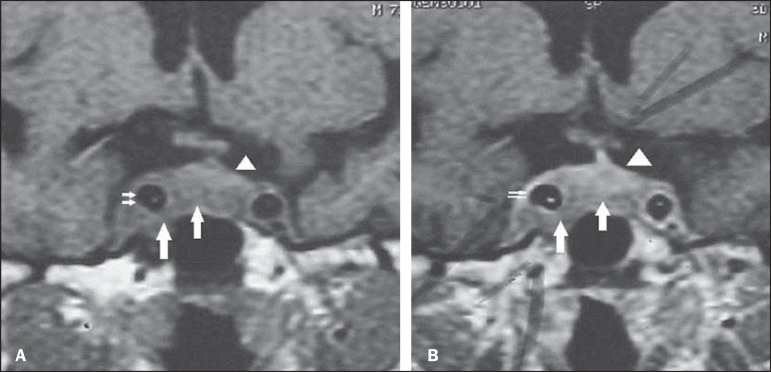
Pre-resection coronal magnetic resonance imaging T1-weighted sequence, before
